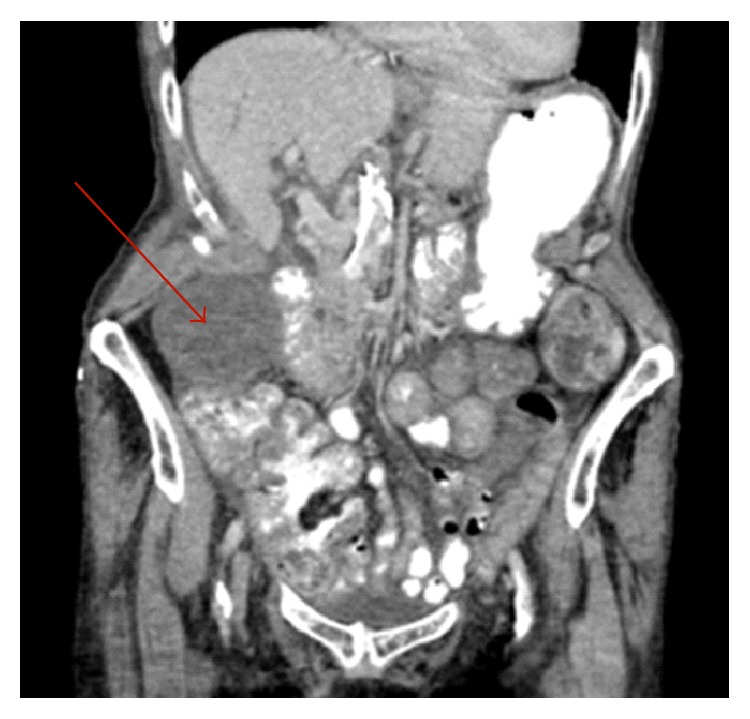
CT scan abdomen and pelvis. Image showing a large “fluid collection” in the right lower quadrant of the abdomen.
